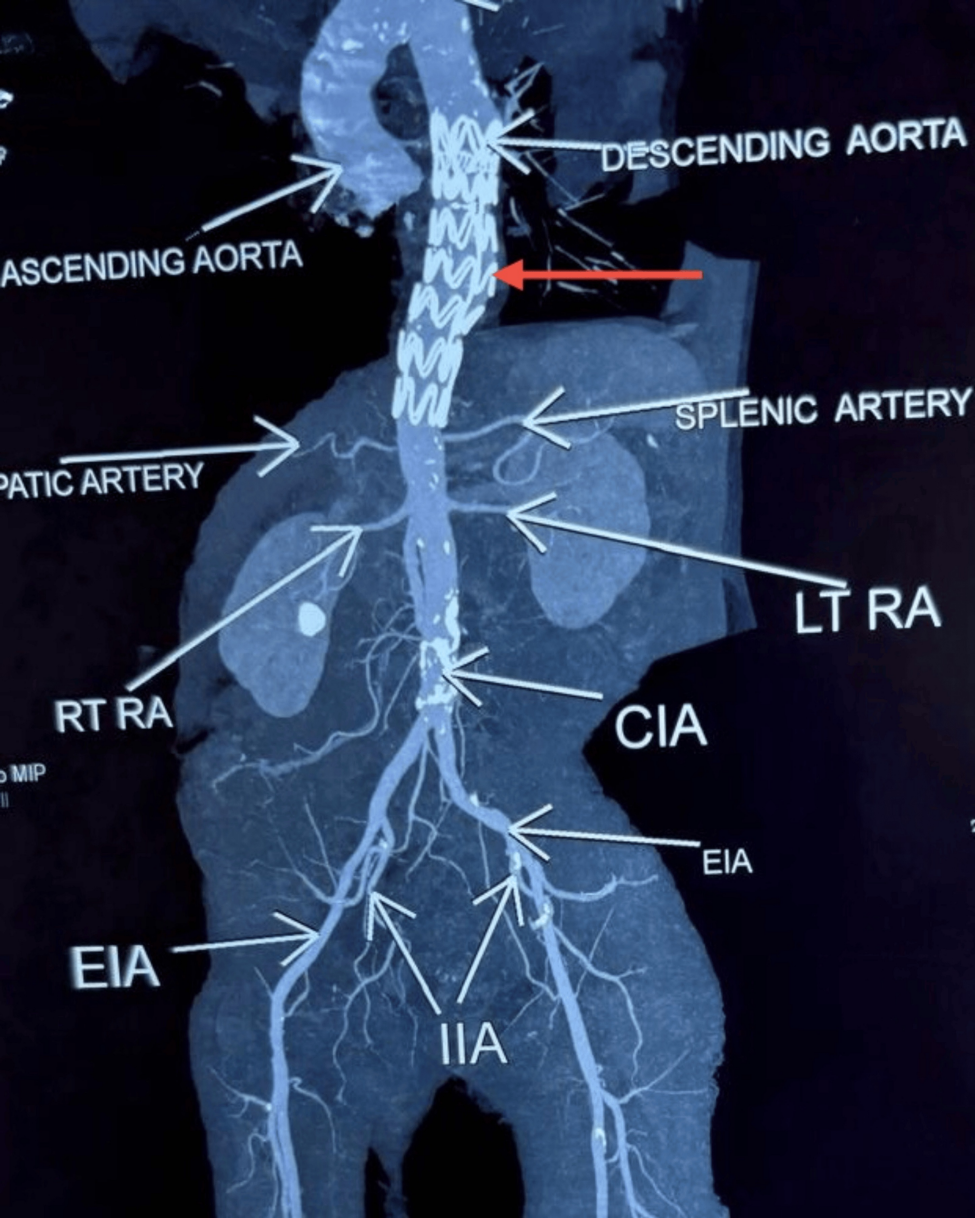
Six-month post-TEVAR CT aortogram showing the Ankura stent graft in the descending thoracic aorta (red arrow) with no evidence of endoleak, graft migration, or recurrent aneurysmal dilatation. The scan confirms stable graft position and preserved perfusion of major visceral and lower limb vessels. TEVAR, thoracic endovascular aortic repair; EIA, external iliac artery; CIA, common iliac artery; RT RA, right renal artery; LT RA, left renal artery
